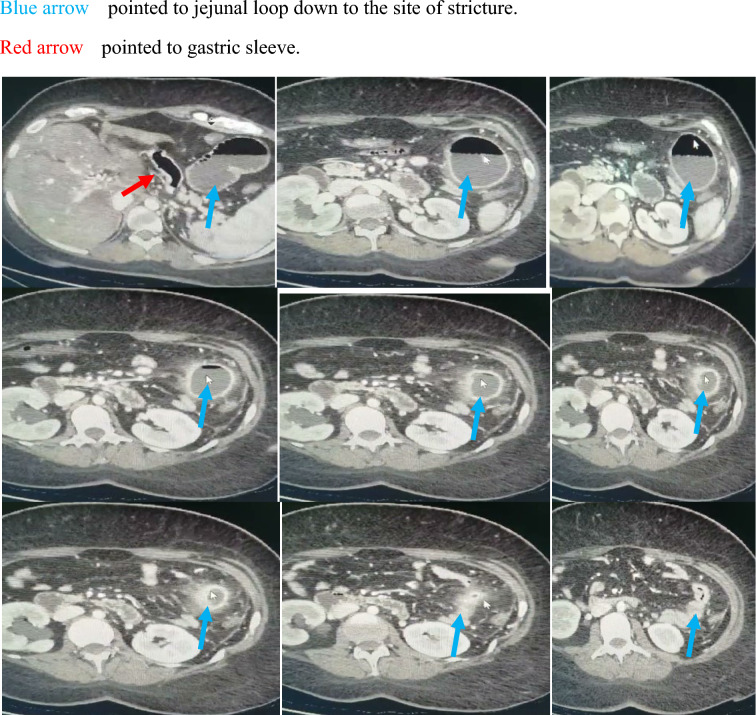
CT evaluation revealed, markedly dilated jejunal loop down to the site of jejunal stenosis (Color figure online)
